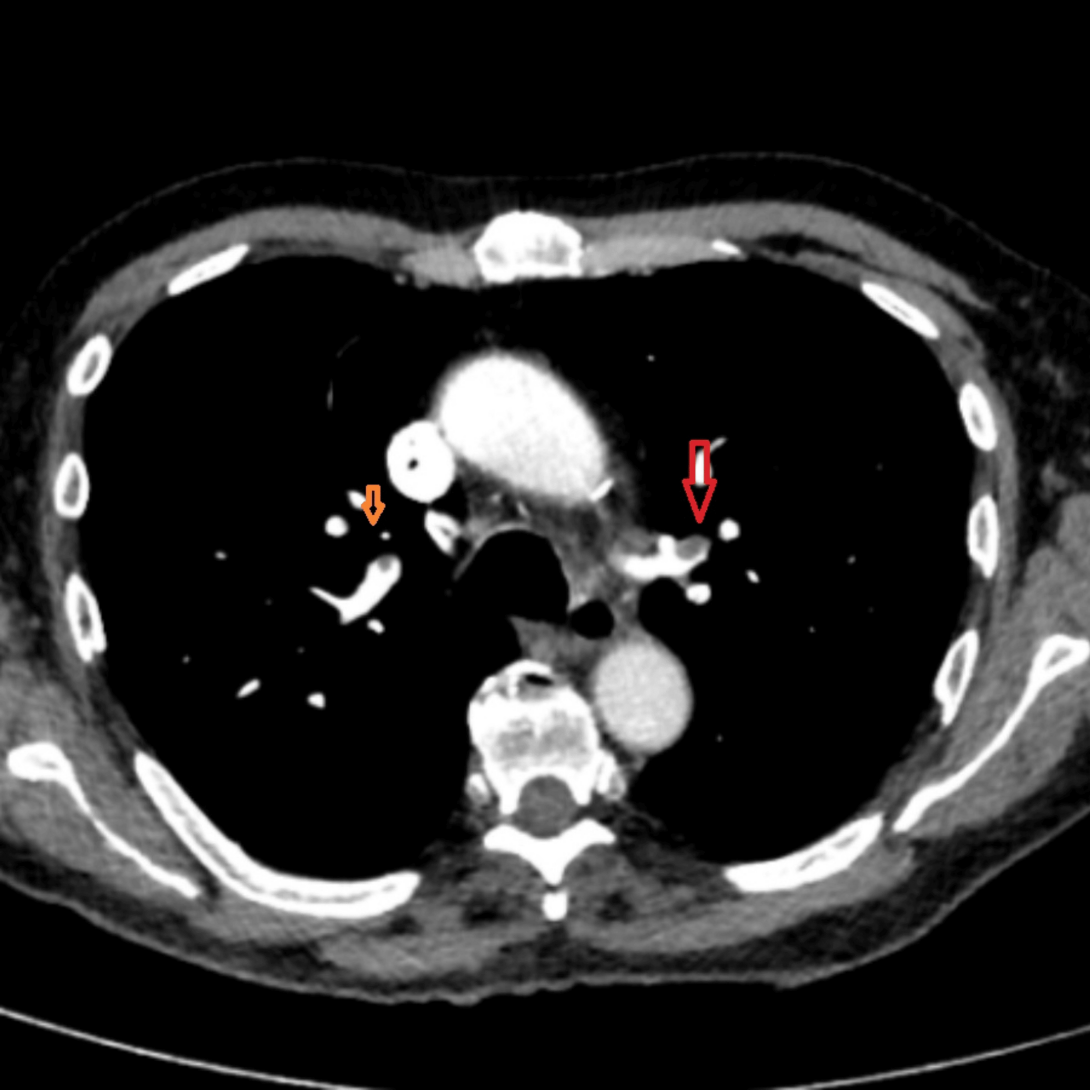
CT pulmonary angiogram showing pulmonary emboli in the right and left pulmonary arteries
